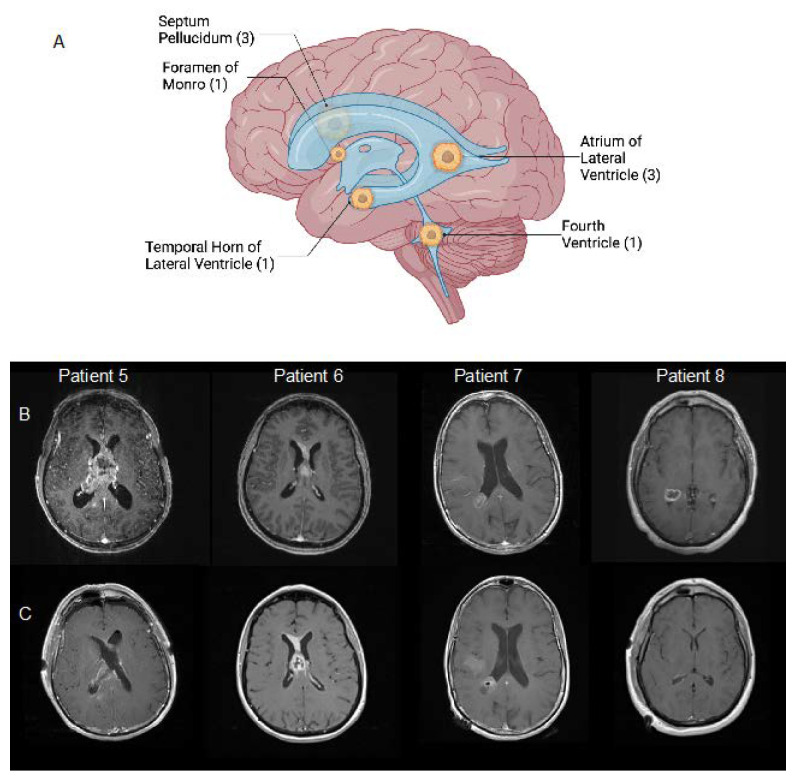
Locations of IVGBM in our cohort created with Biorender.com (A). T1 post-contrast magnetic resonance images showing (B) IVGBM prior to resection and (C) postoperatively in 4 patients with available radiographic images. Patients 5 and 7 received subtotal resection. Patient 6 received biopsy. Patient 8 received gross total resection.
